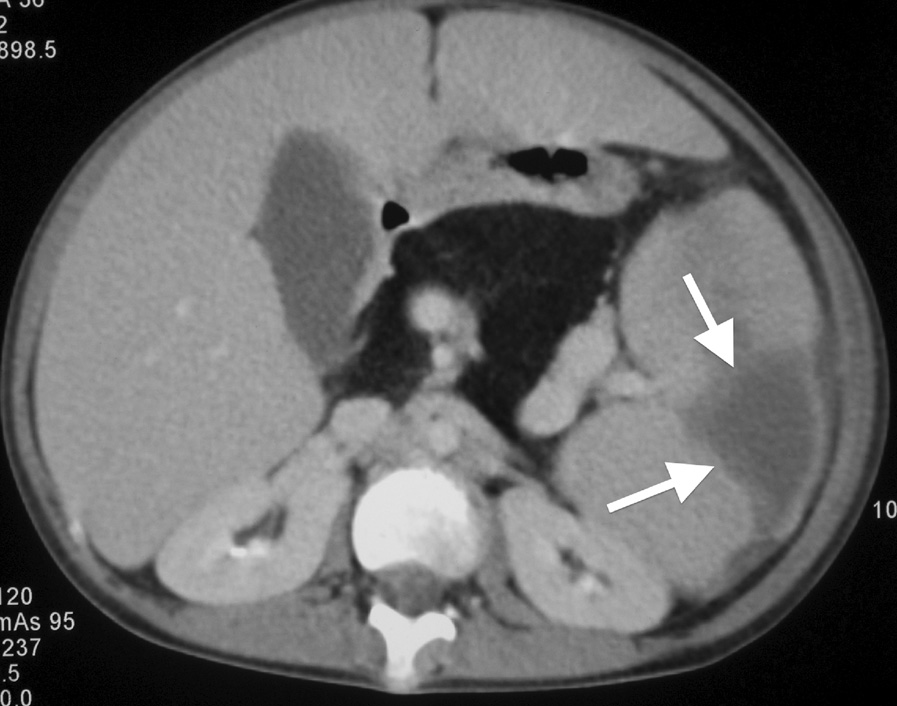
Contrast enhanced computed tomography (CT) of abdomen. The spleen is enlarged and contains a well-defined wedge shaped peripheral zone of low attenuation which does not enhance consistent with infarction (arrows). There is enlargement and tortuosity of the splenic vein. The pancreas is enlarged and of uniform low attenuation consistent with fatty replacement.
